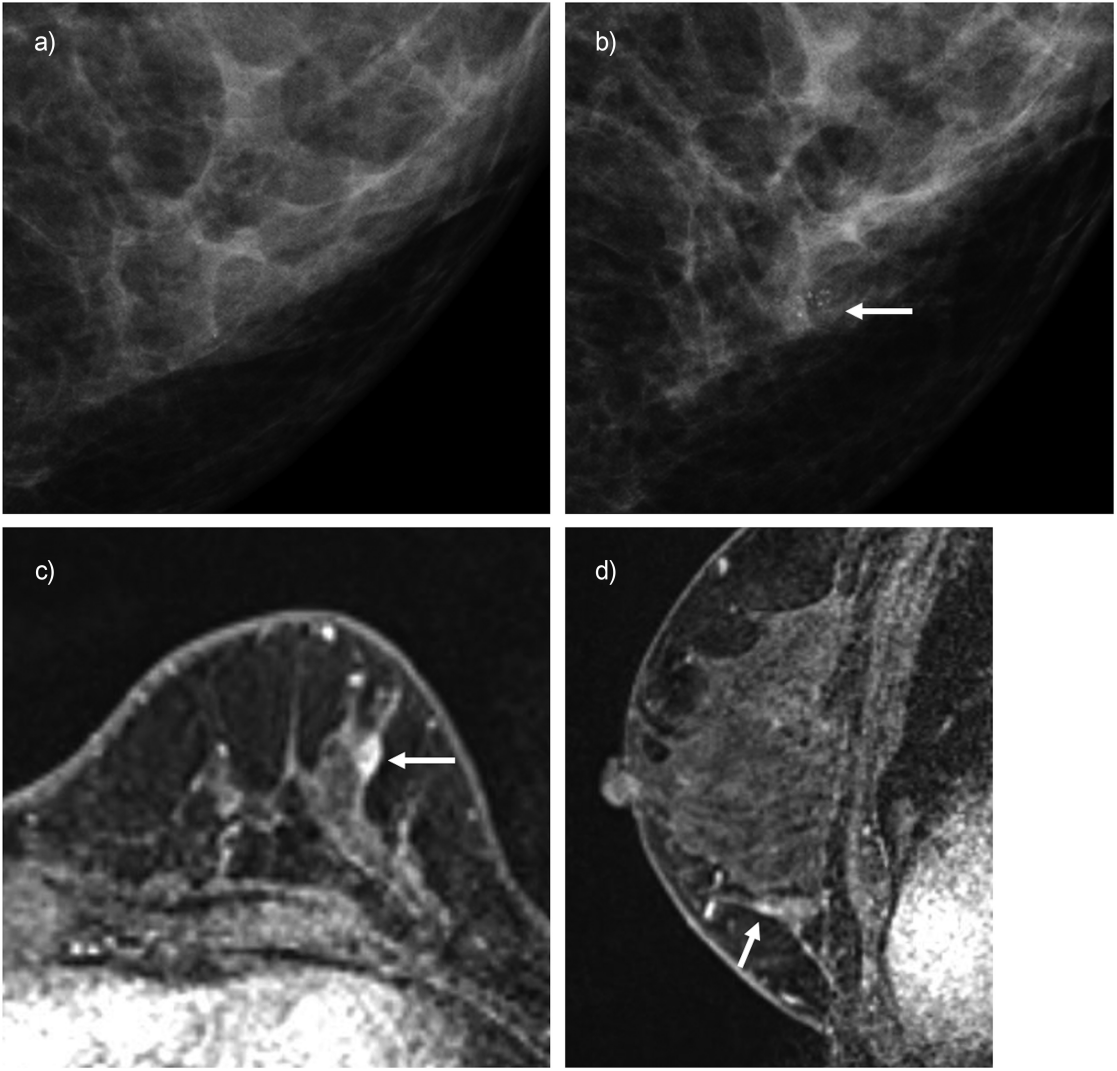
BRCA2* carrier of pathogenic variants with a right breast-conserving surgery at her 40s. The woman started imaging screening with yearly mammography 14 years after her surgery. (a) and (b) are the mediolateral oblique mammography images. (a) Mammogram was assessed as normal 1 year before the lesion was suspected. (b) A clustered calcification appeared in the lower outer quadrant area. (c) and (d) are the high-resolution DCE-MRI with axial and sagittal images. A 5-mm enhancing lesion was detected and described as BI-RADS Category 4A. The lesion was biopsied and showed DCIS, diagnosed 22 years after she got her first cancer. The white arrows in each image indicate the lesion. BI-RADS, Breast Imaging Reporting and Data System; DCE-MRI: dynamic contrast-enhanced MRI; DCIS, ductal carcinoma *in situ*.
